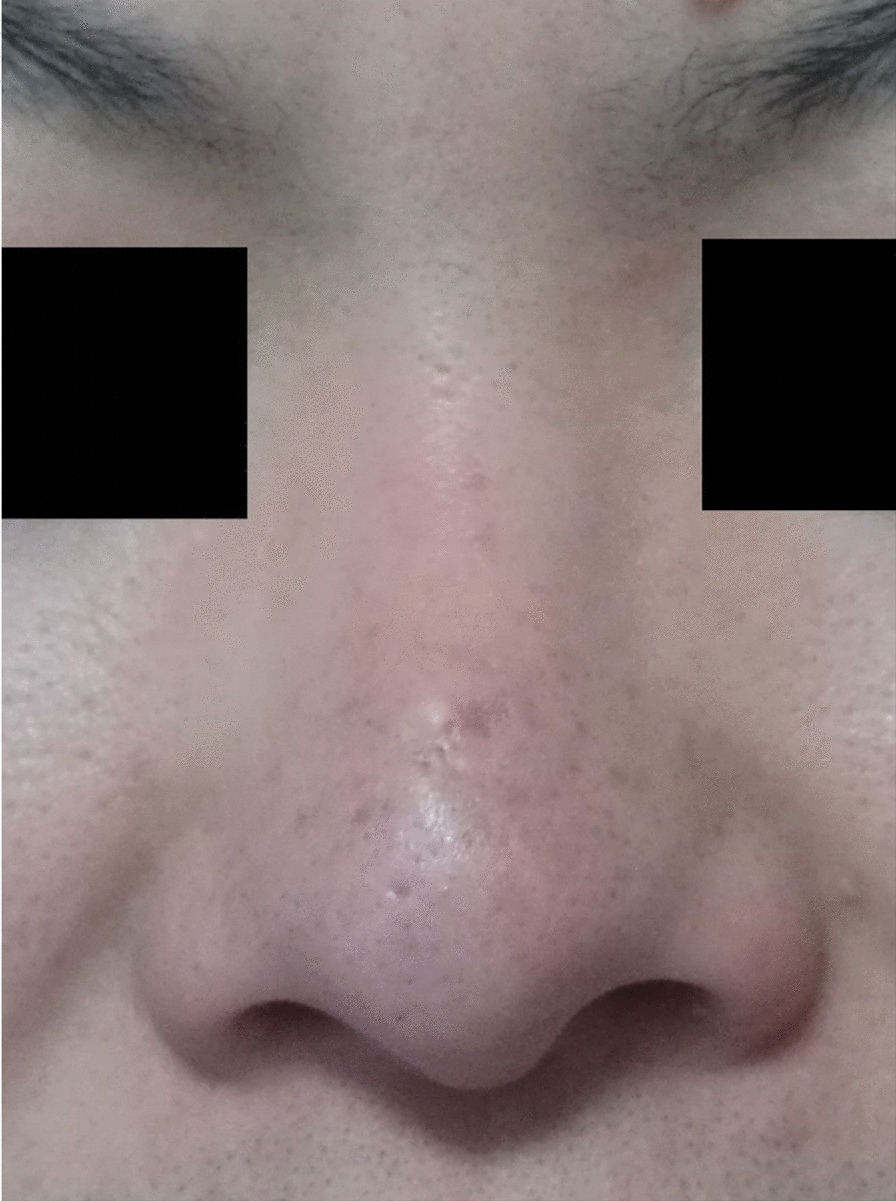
Postoperative picture of the patient (4 years). CT showing the extent of the sinus with in it
